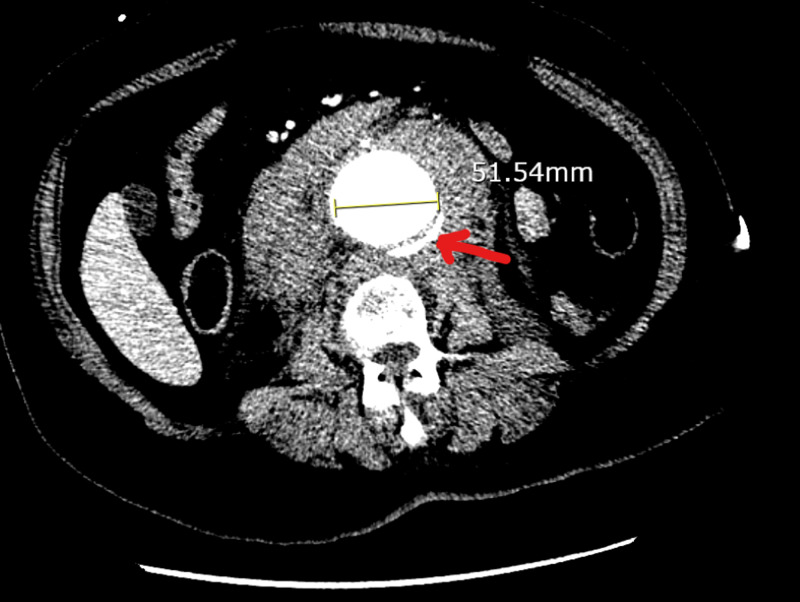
A CTA abdomen and pelvis showing a 5.1 cm AAA with a high-attenuating crescent sign (red arrow) CTA: computed tomography angiogram; AAA: abdominal aortic aneurysm
